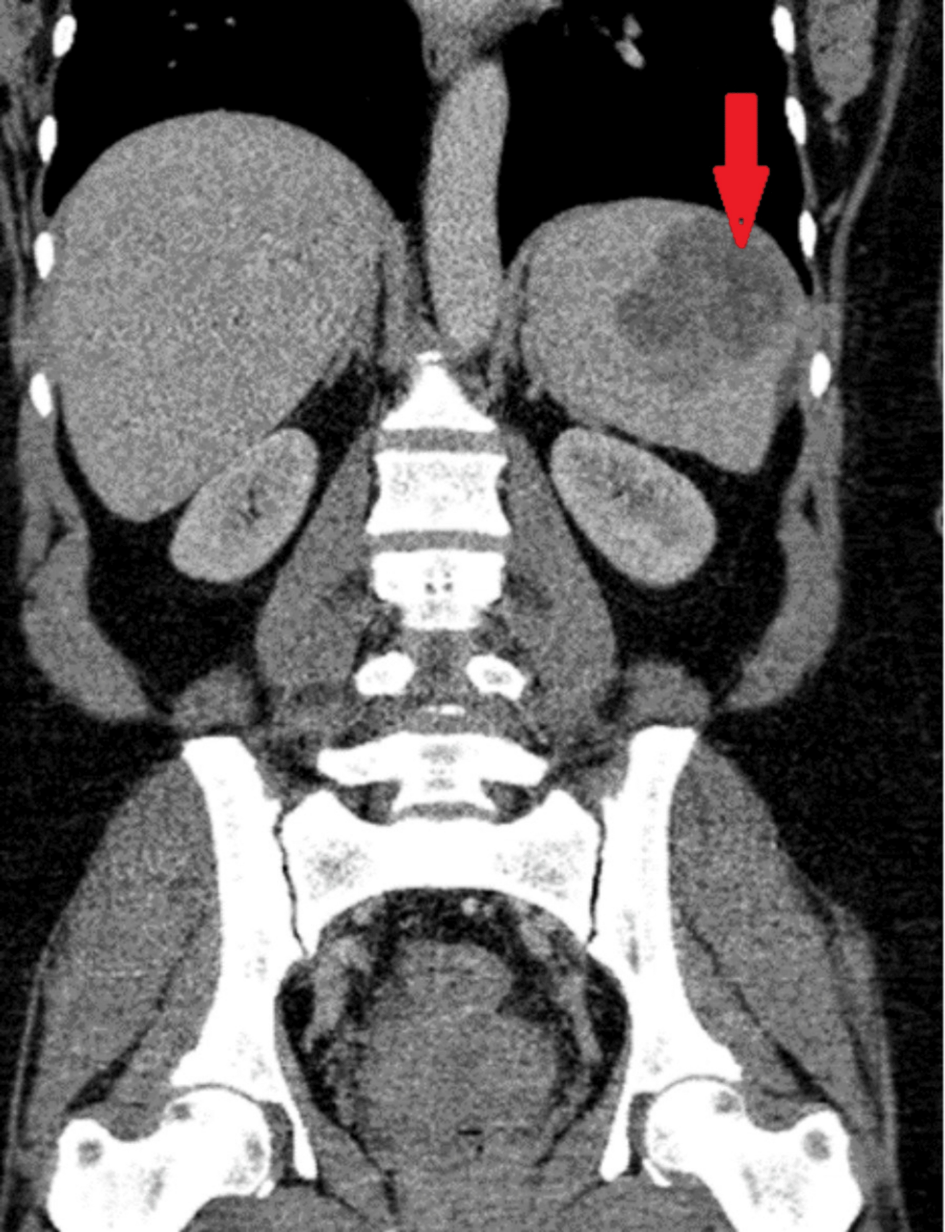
CT abdomen with IV contrast-portal phase, in coronal plane, showed splenic, subcapsular, heterogenous predominantly hypodense lesion with internal streak enhancing components (red arrow).
